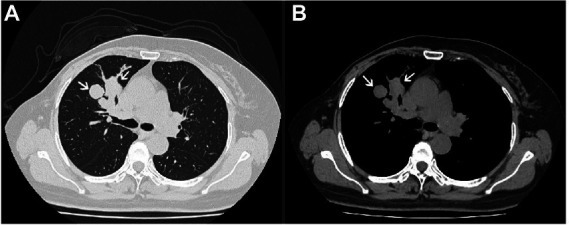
Chest computed tomography showing a soft-tissue density mass in the upper lobe of the right lung. (A) lung window; (B) mediastinal window.
